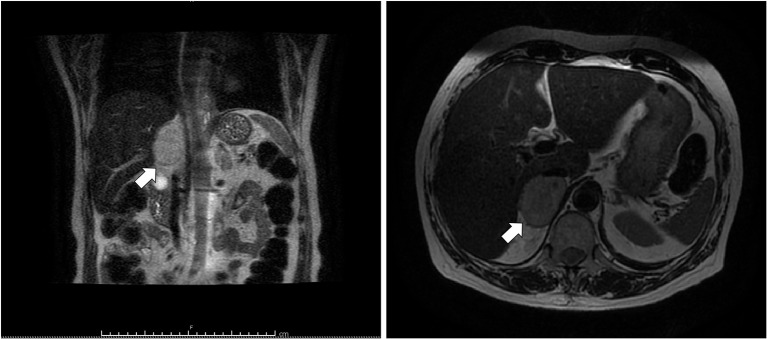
T2-weighted MRI of abdomen. Coronal (left) and axial (right) planes showing a hyperintense right adrenal mass (arrow) measuring 50 × 53 mm.
